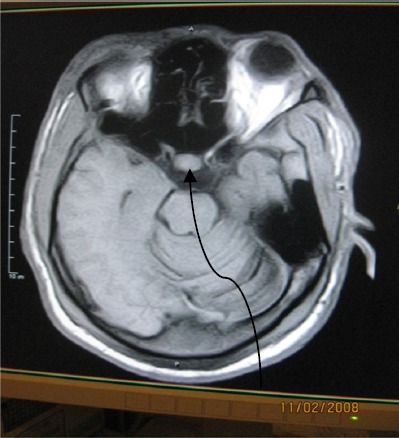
Axial MRI T1W image showing pituitary microadenoma, atrophic left temporal lobe with small tempoal fossa and enlarged mastoid air cell
